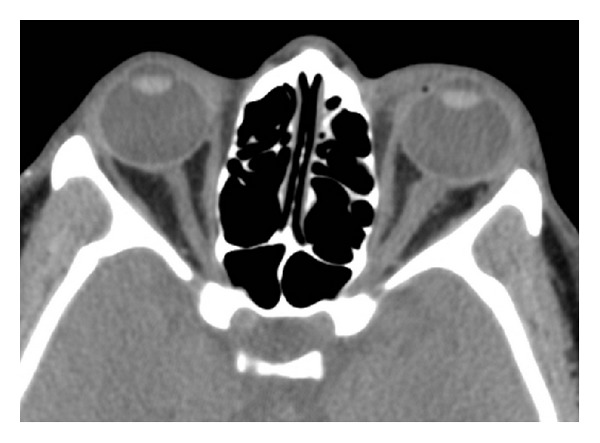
CT orbits demonstrating irregularity to left optic nerve and adjacent fat stranding. Unenhanced CT orbit study demonstrates shaggy irregularity of the distal left optic nerve, which is enlarged compared to the right. Surrounding mild fat stranding is also evident. Minor preseptal soft tissue swelling is apparent with tracking of a thin hematoma along the lateral left orbital wall. The globes are intact, and no additional traumatic injury is visualized.
